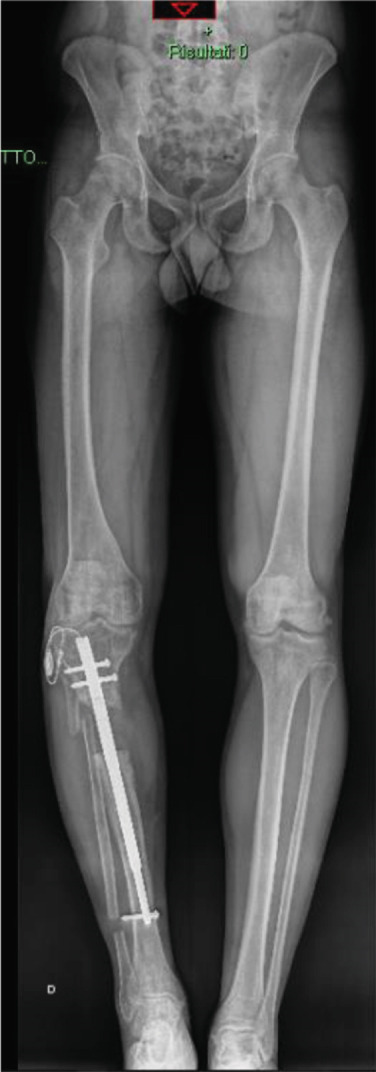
Radiograph showing progressive lengthening with motorized intramedullary lengthening nail.
